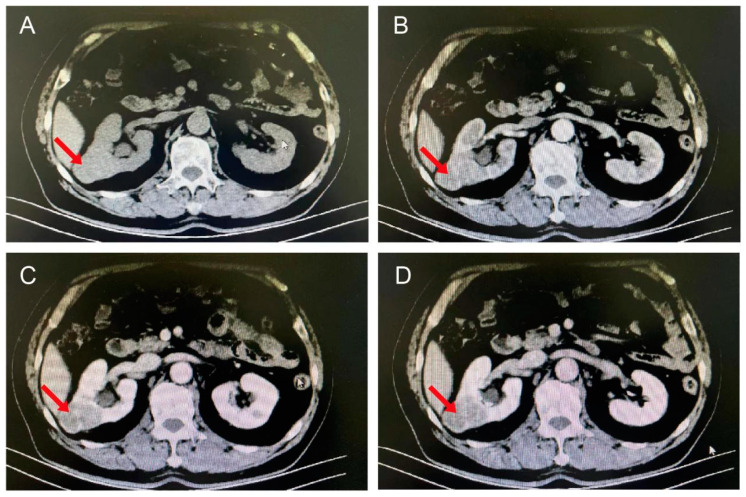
Enhanced CT images of the kidneys. The figure indicates a 4.2 × 3.5 × 4 cm nodule in the middle part of the right kidney, with dilatation and fluid accumulation in the right renal pelvis, calyces, and the upper segment of the ureter. (A) Unenhanced. A solitary lobulated mass is present within the right kidney parenchyma, protruding beyond the renal contour. The mass has uneven density, with irregular low-density areas inside and unclear margins. (B) Enhanced arterial phase. The mass shows obvious non-homogeneous enhancement, with its density close to that of the renal cortex, and a non-enhanced necrotic area visible in the center. (C,D) Enhanced portal venous phase and enhanced venous phase. The mass demonstrates rapid delineation, with a density lower than that of the normal renal parenchyma. The red arrow points to the kidney cancer.
